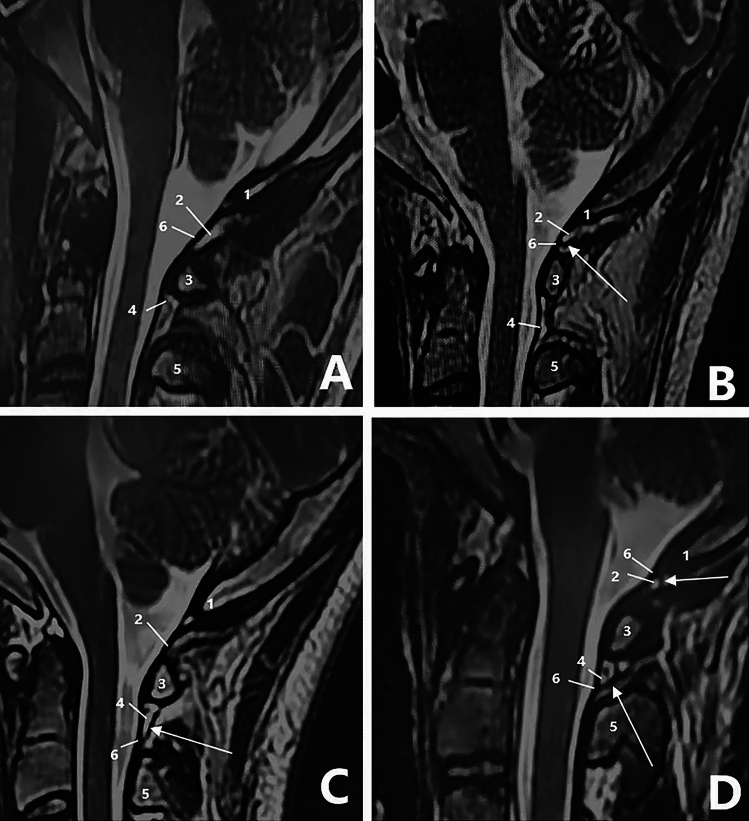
The T2-weighted imaging image of the occipital and cervical portions (sagittal view) 1, occipital bone; 2, posterior atlanto-occipital interspace (PAOiS); 3, posterior arch of atlas; 4, posterior atlanto-axial interspace (PAAiS); 5, spinous process of axis; 6, upper cervical dura mater. Arrows indicate the myodural bridge (MDB). Figure A: Type A MDB complex (MDBC): no MDBC low-signal shadow connected to the dura mater in either the PAOiS or PAAiS, Figure B: Type B MDBC: MDBC low-signal shadow connected to the dura mater in the PAOiS only (arrow), Figure C: Type C MDBC: MDBC low-signal shadow connected to the dura mater in the PAAiS only (arrow), and Figure D: Type D MDBC: both the PAOiS and PAAiS show MDBC hyposignal shadow with dural connection (arrow)
